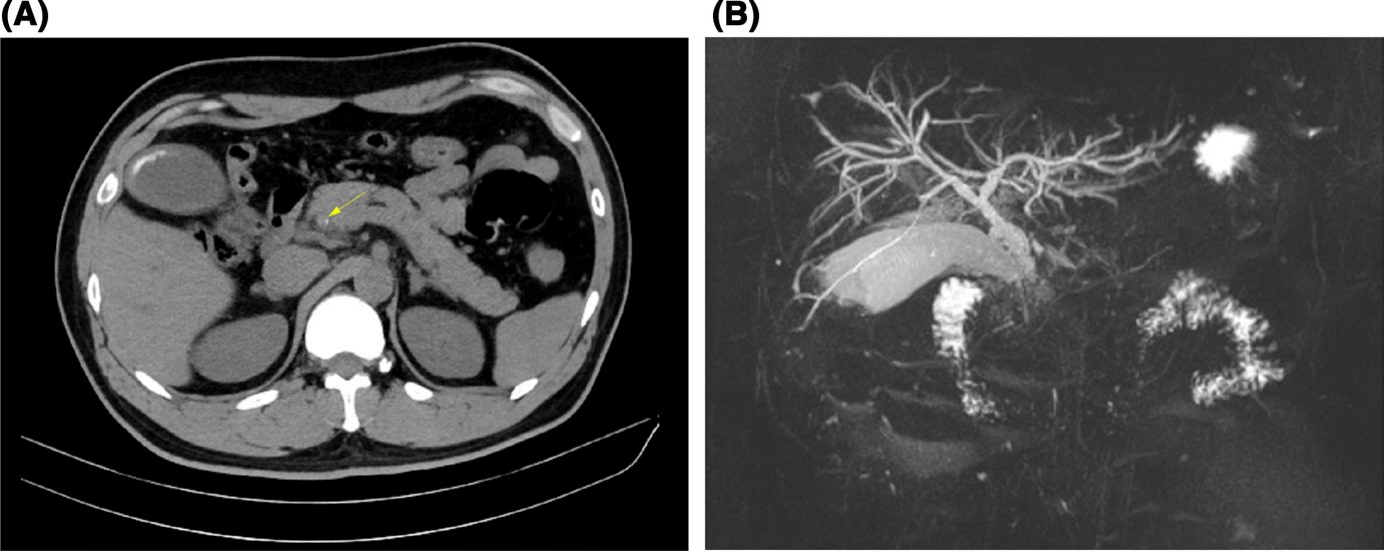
Preoperative imaging. (A) Computed tomography of the gallbladder and common bile duct stones (arrow); (B) Magnetic resonance cholangiopancreatography showing slight dilation of the intrahepatic bile duct and the unclear lower part of the common bile duct
